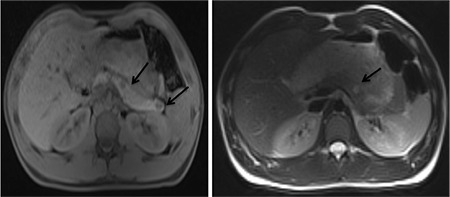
Non-contrast, T1-weighted axial pancreas magnetic resonance imaging (MRI) showing hypointense lesions (black arrows) in the tail and body of the pancreas (A). T2-weighted axial MRI revealing a hyperintense lesion (black arrow) in the body of the pancreas (B)
